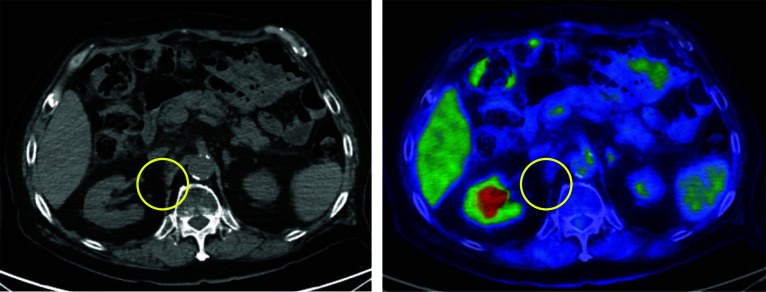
Positron emission tomography-computed tomography findings after chemotherapy. There was no evidence of tumor recurrence, and the condition was diagnosed as clinical complete response (yellow circle)
